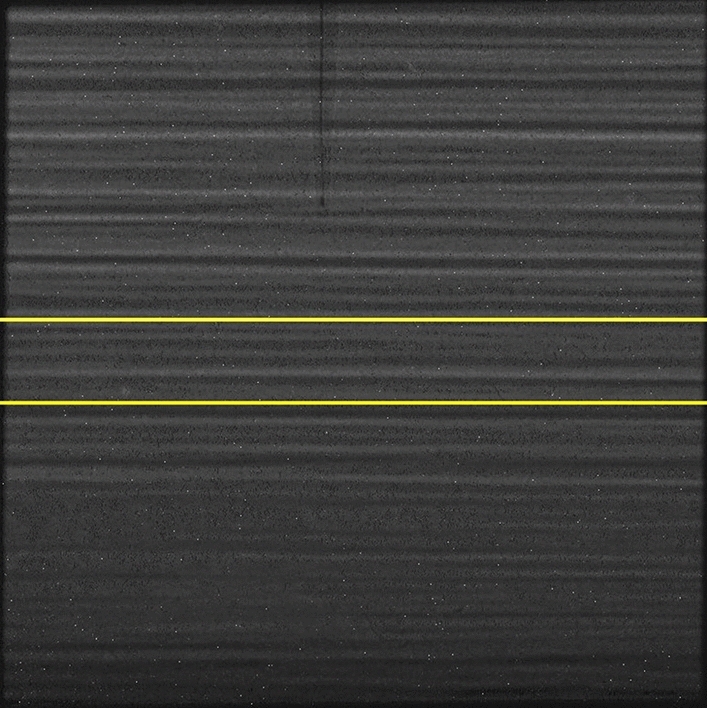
X-ray transmission image of a 5 mm $$\times$$ 5 mm irradiated region and the hydrogen probe tip centrally above it. Images such as this were used to determine the distance from the probe tip to the irradiated region. Yellow lines outline the region that was irradiated. Figure made with an image processed by FIJI^56^.
